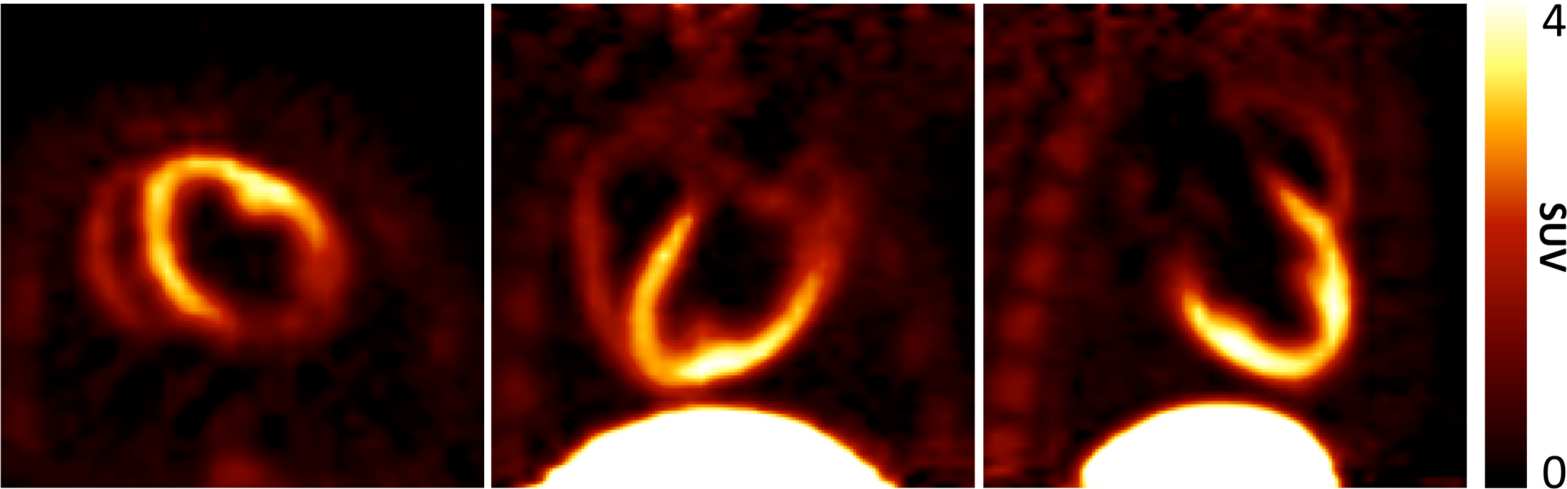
Typical ^18^F-TPP^+^ SUV image, integrated from 60–120 minutes after IV bolus injection. SUV is highest in liver, followed by LV myocardium, with lower activity in the visible in bone marrow.
